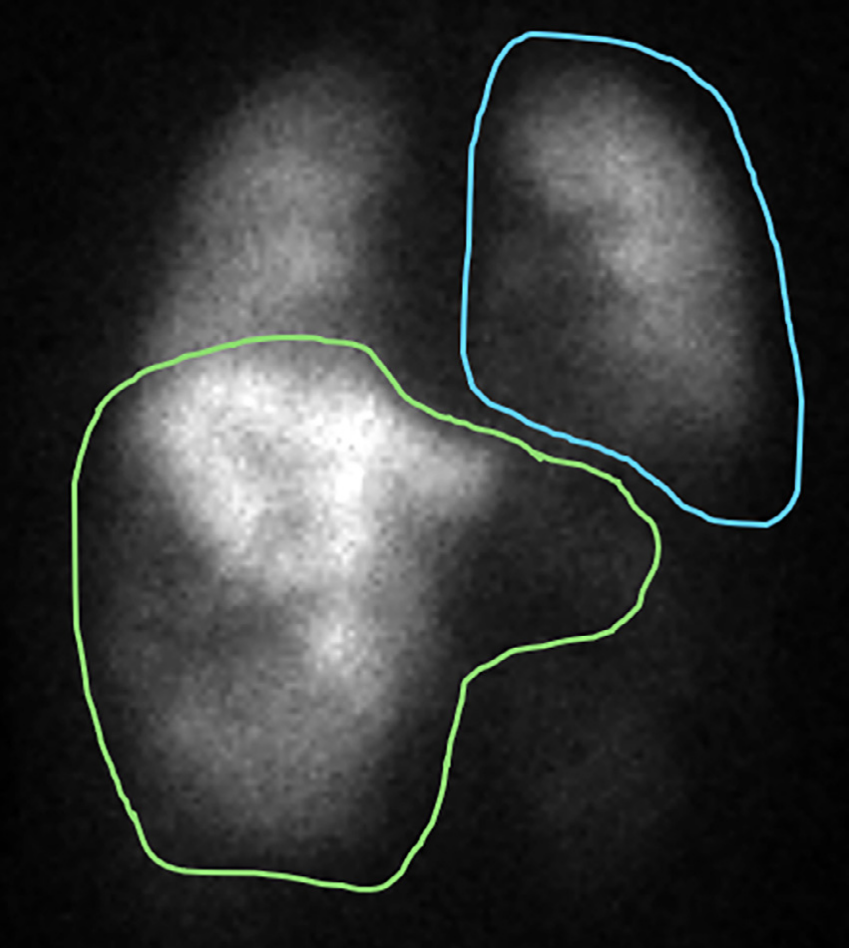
Planar scintigraphy after initial mapping with 99Tc-MAA demonstrated substantial radiotracer uptake within the liver as well as the lungs. The calculated LSF was 25%. Green: Liver. Blue: Lung.
